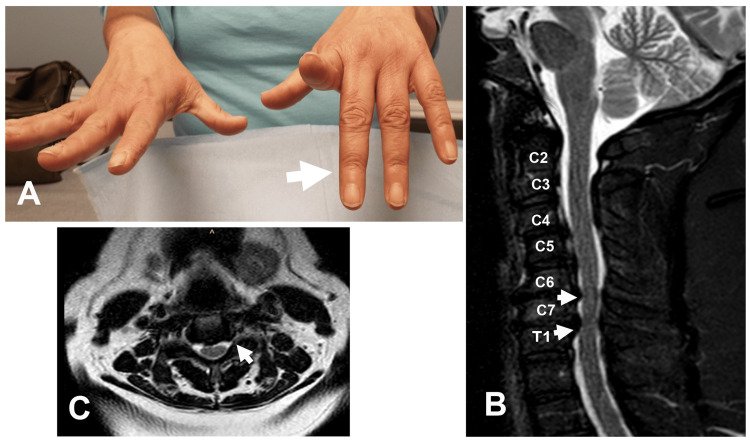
Partial Finger Drop Caused by Cervical Radiculopathy (A) Partial finger drop involving digits 3, 4, and 5 of the left hand (arrow). MRI of the (B) sagittal view showing cervical spondylosis at C6-7 and C7-T1 (arrows) and (C) axial view at C6-7 showing left foraminal stenosis at C6-7 (arrow).
